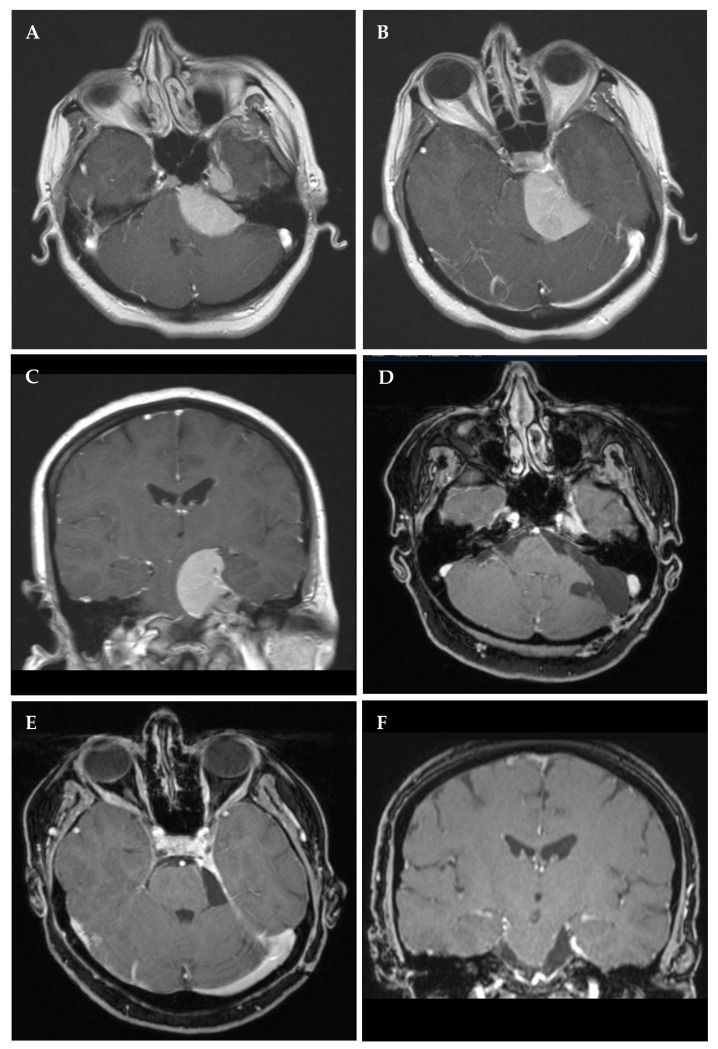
Left petroclival meningioma in a 48-year-old female with headaches and intermittent dysphagia. Preoperative contrast-enhanced T1 magnetic resonance imaging (MRI) in axial (A,B) and coronal I (C) reconstructions with compression of the brain stem but without invasion of the cavernous sinus. Most of the lesion was situated in the infratentorial compartment and was reached by a retrosigmoid approach, with only minor residual tumor appreciated in Meckel’s cave (D–F). Six years later, the patient underwent radiation therapy (54 Gy) for tumor progression, while she remained without deficits throughout follow-up.
